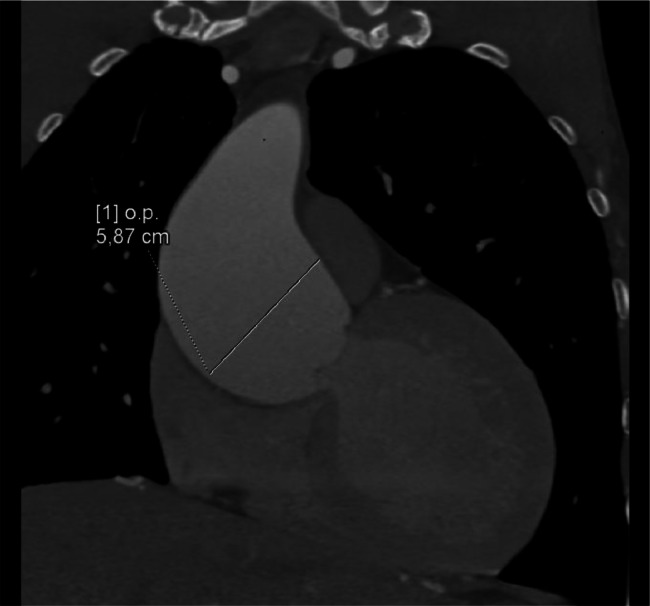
Coronal reconstruction CT image highlighting the aneurysmal ascending aorta.
